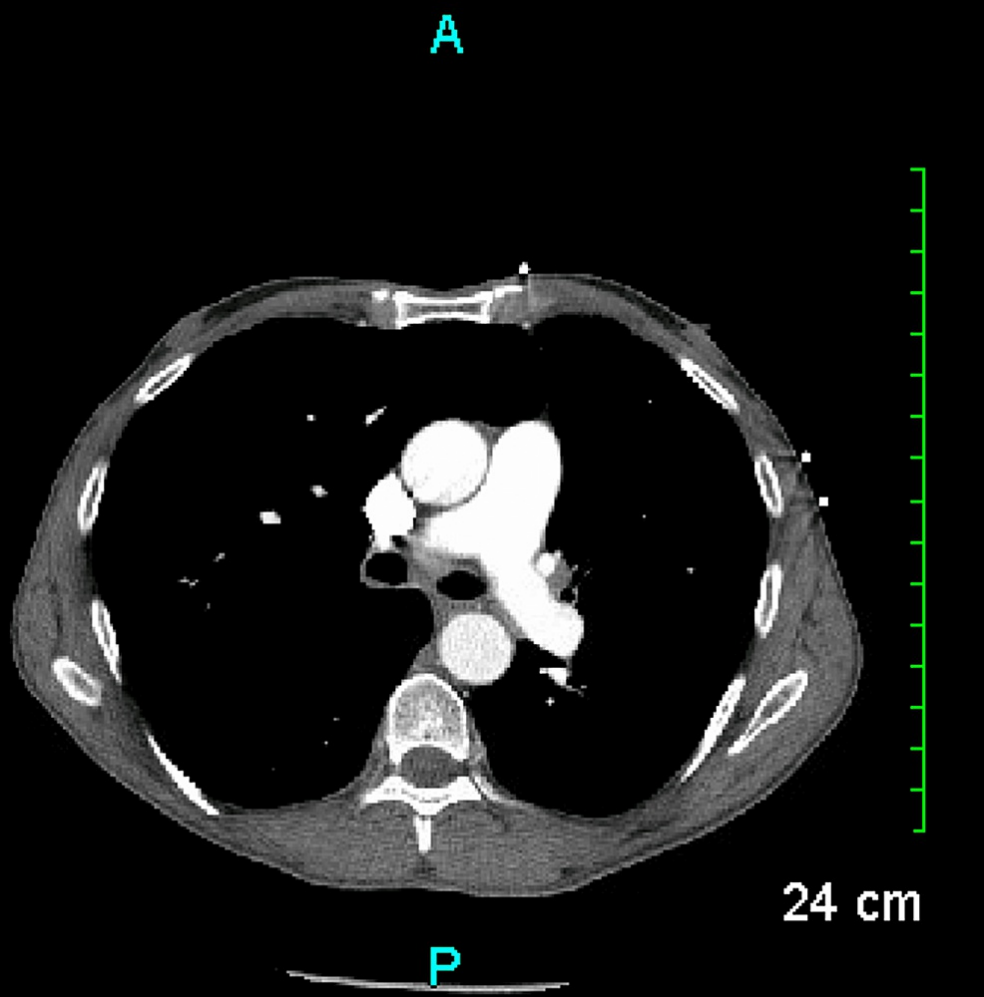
CT angiography negative for pulmonary embolism
